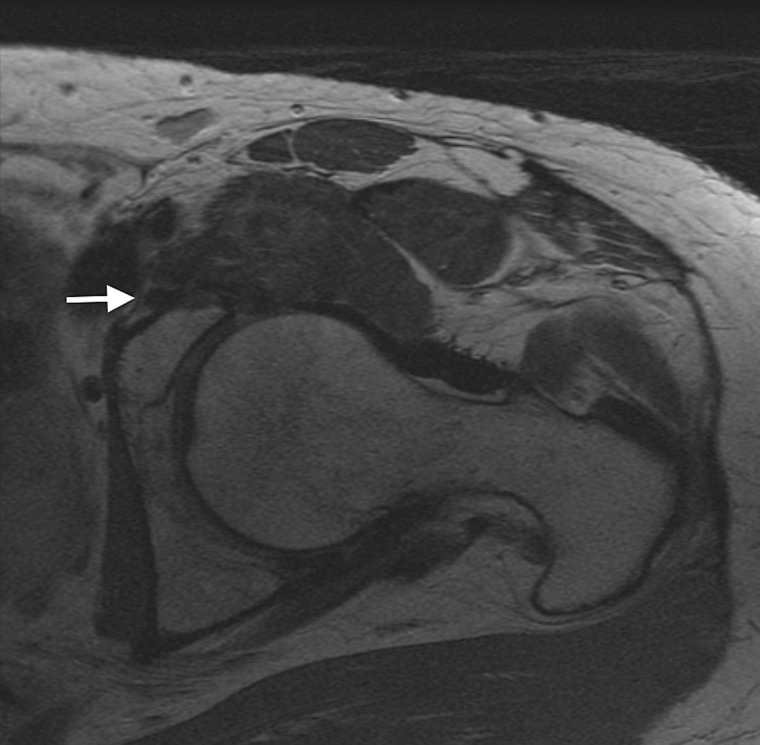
Axial MRI displaying anchor material (white arrow) perforating through the medial cortex, adjacent the neurovascular bundle 68 × 67 mm.
